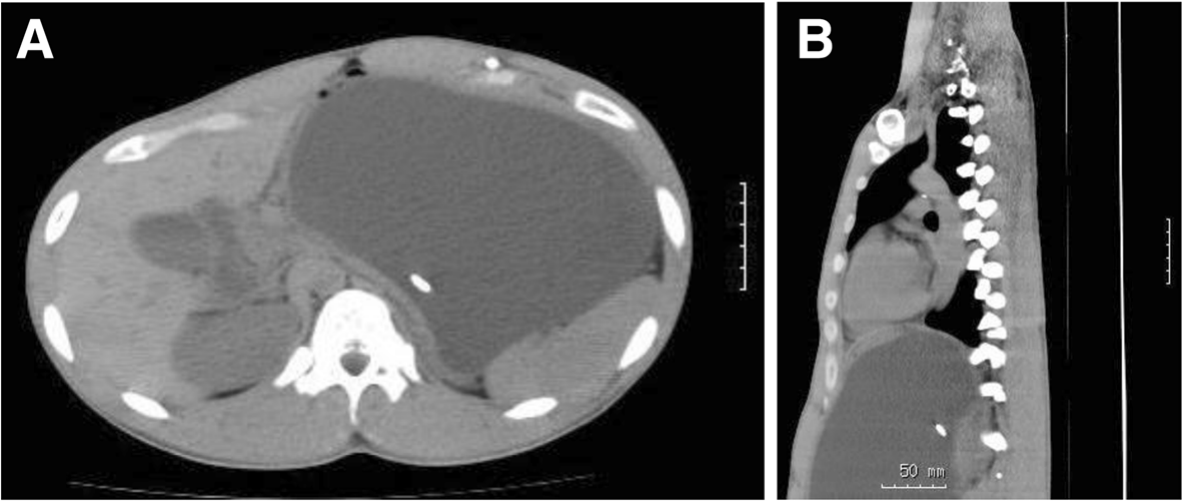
Abdoinal CT (A: axial, B: sagital) showing the fluid collection adjacent to the VPS catheter tip. A,B Abdominal CT showing the fluid collection adjacent to the VPS catheter tip.
